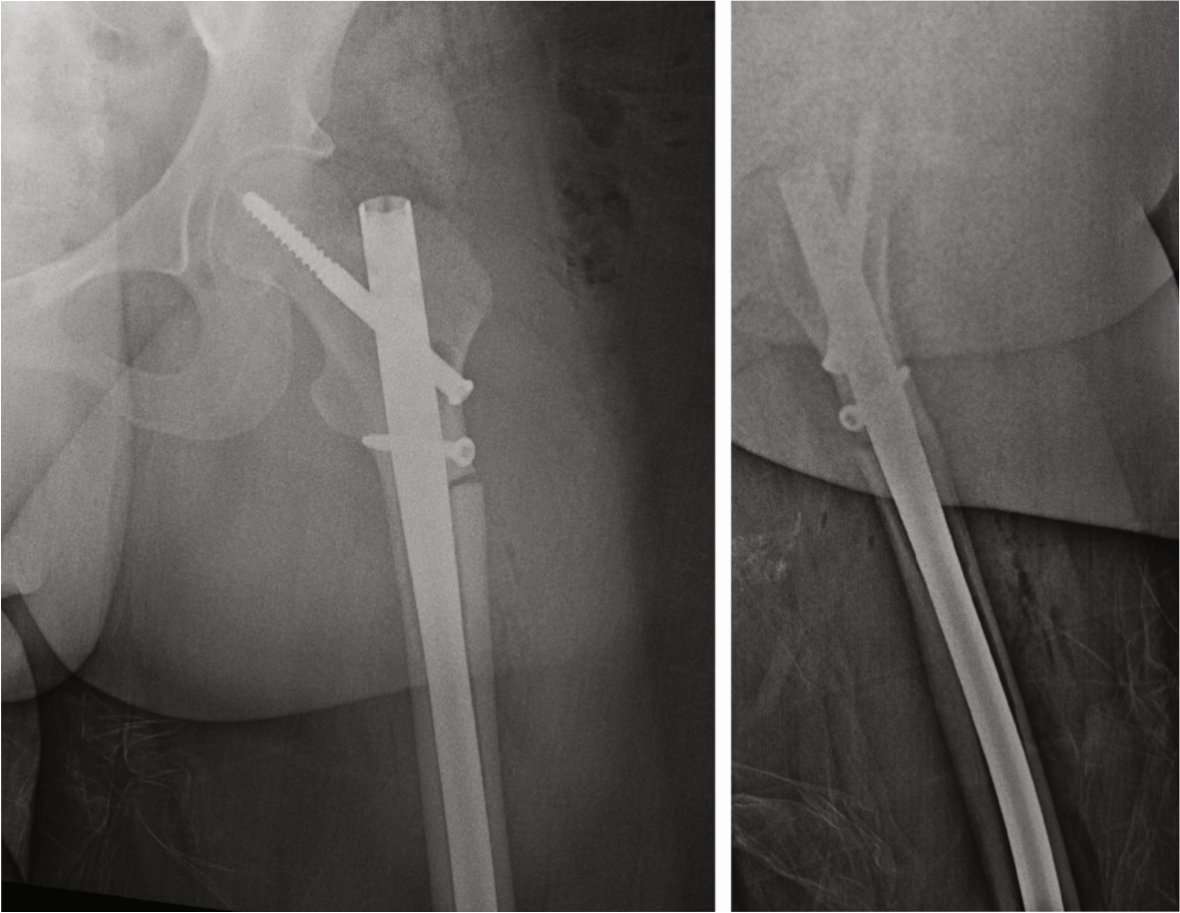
Anteroposterior and lateral radiograph of the left femur demonstrating internal fixation of the subtrochanteric atypical fracture.
